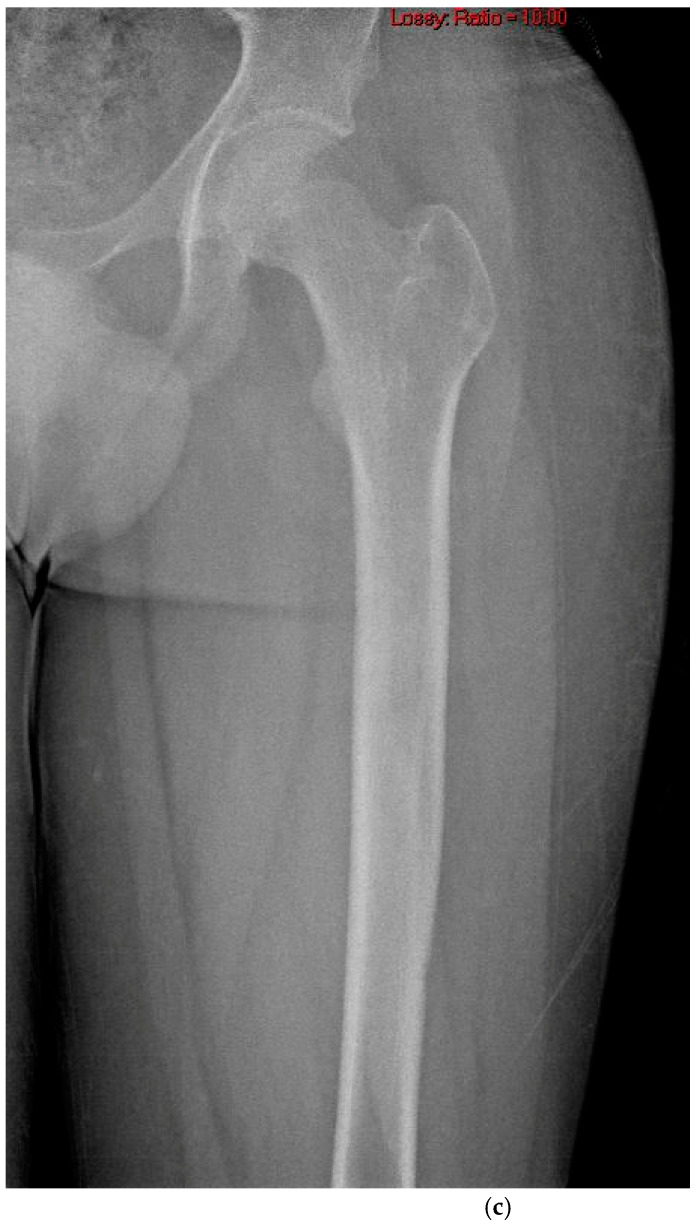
A 12.5-year-old with a subtrochanteric fracture with extension into the proximal femur. (a) Emergency room radiograph demonstrating a left subtrochanteric fracture with proximal extension. (b) Immediate postoperative radiograph with the uniplanar external fixator and two elastic intramedullary nails (ESINs). (c) After removal of the ESINs, with full fracture healing.
